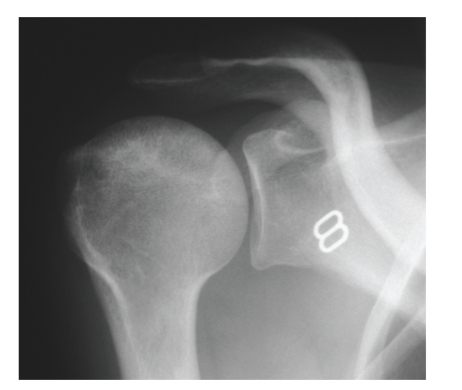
Plain radiograph of the shoulder showing early patchy sclerosis of the right humeral head consistent with early avascular necrosis (snow storm appearance).
